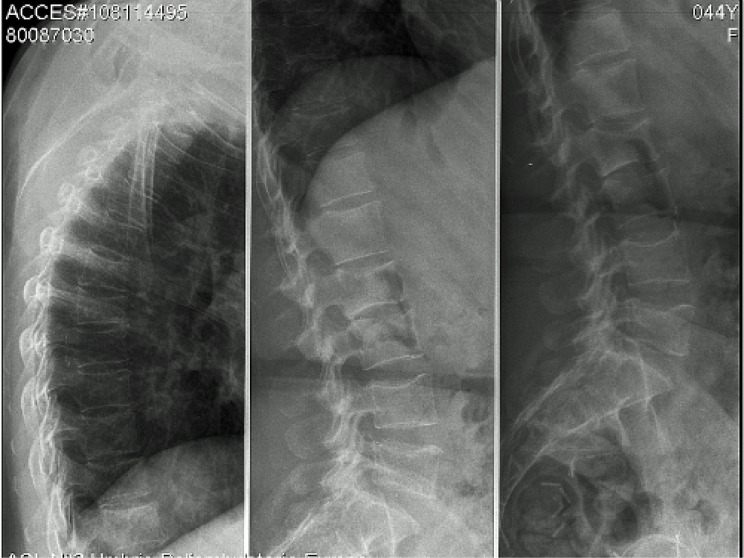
Dorso-lumbosacral spine X-ray: fracture deformities of D/7and of the bodies of L/2-4, in a general aspect of diffuse osteopenia.
